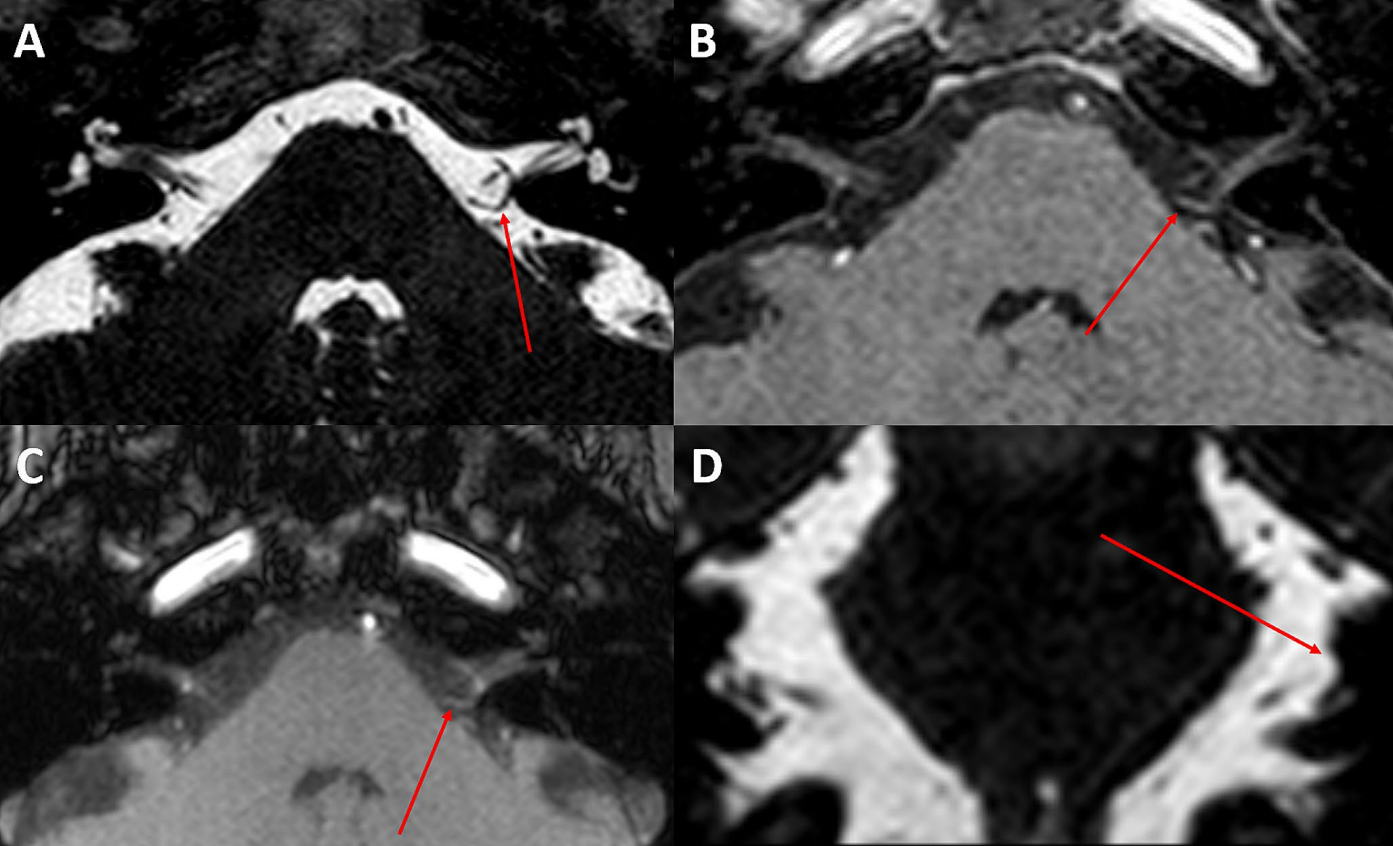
Brain MRI. (A) Axial T2 FIESTA, (B) T1-weighted post-gadolinium volumetric, (C) axial time-of-flight (TOF), (D) coronal T2 FIESTA sequences, showing a possible NVC between an abnormal loop of the AICA and the VIII cranial nerve (red arrow). In our case, there was a concordance between symptoms (mainly the left tinnitus) and the NVC side
